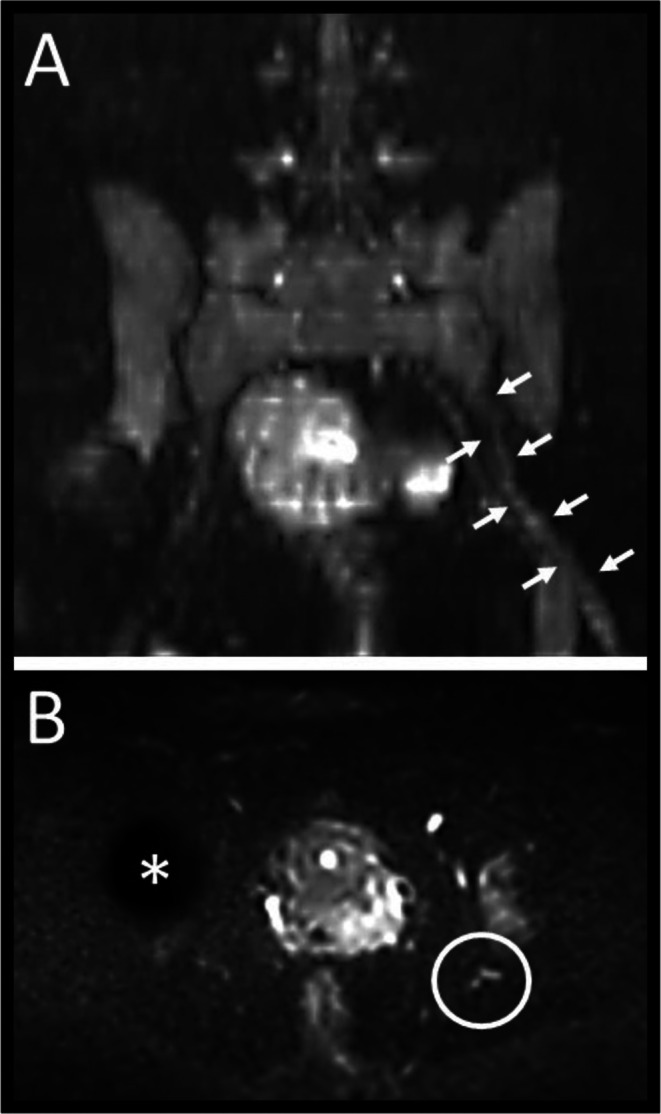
Diffusion‐weighted MRI scans of the lumbar spine showed a thickened and signal‐altered left sciatic nerve in the diffusion weighting (A: Frontal plane, arrows; B: Transversal plane, circle). Star: Artifact due to total hip arthroplasty.
